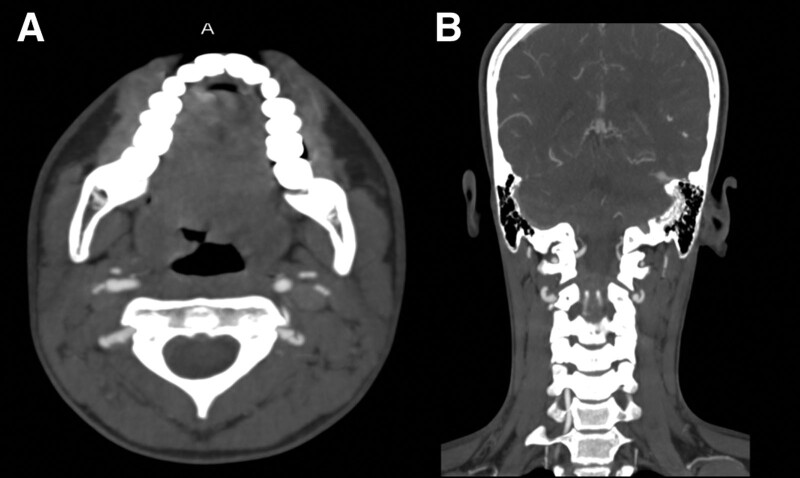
(A and B) Dissecting aneurysm of left vertebral artery: axial and coronal CT angiogram with maximum intensity projection shows small dissecting saccular shaped aneurysm identified at the distal left V3 segment vertebral artery. CT = computed tomography.
